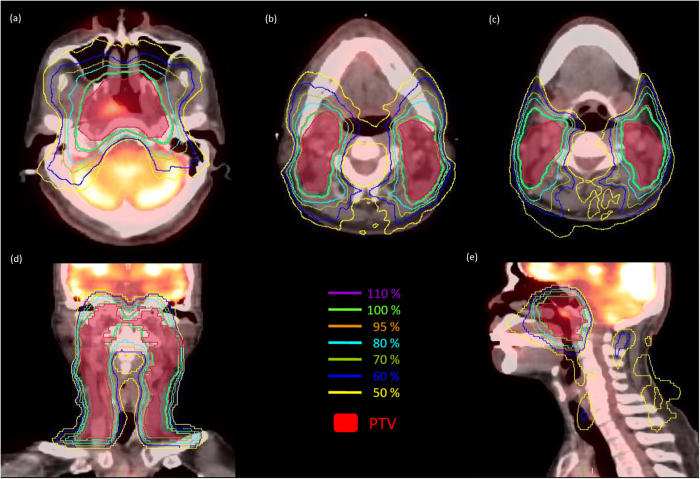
The isodose distributions on transverse, coronal, and sagittal views for one representative nasopharyngeal carcinoma case planned by helical tomotherapy using PET/CT image sets. (a) At the nasopharyngeal region. (b) At the upper neck region near the parotid glands. (c) At the upper neck region near the submandibular glands. (d) Coronal view. (e) Sagittal view.
